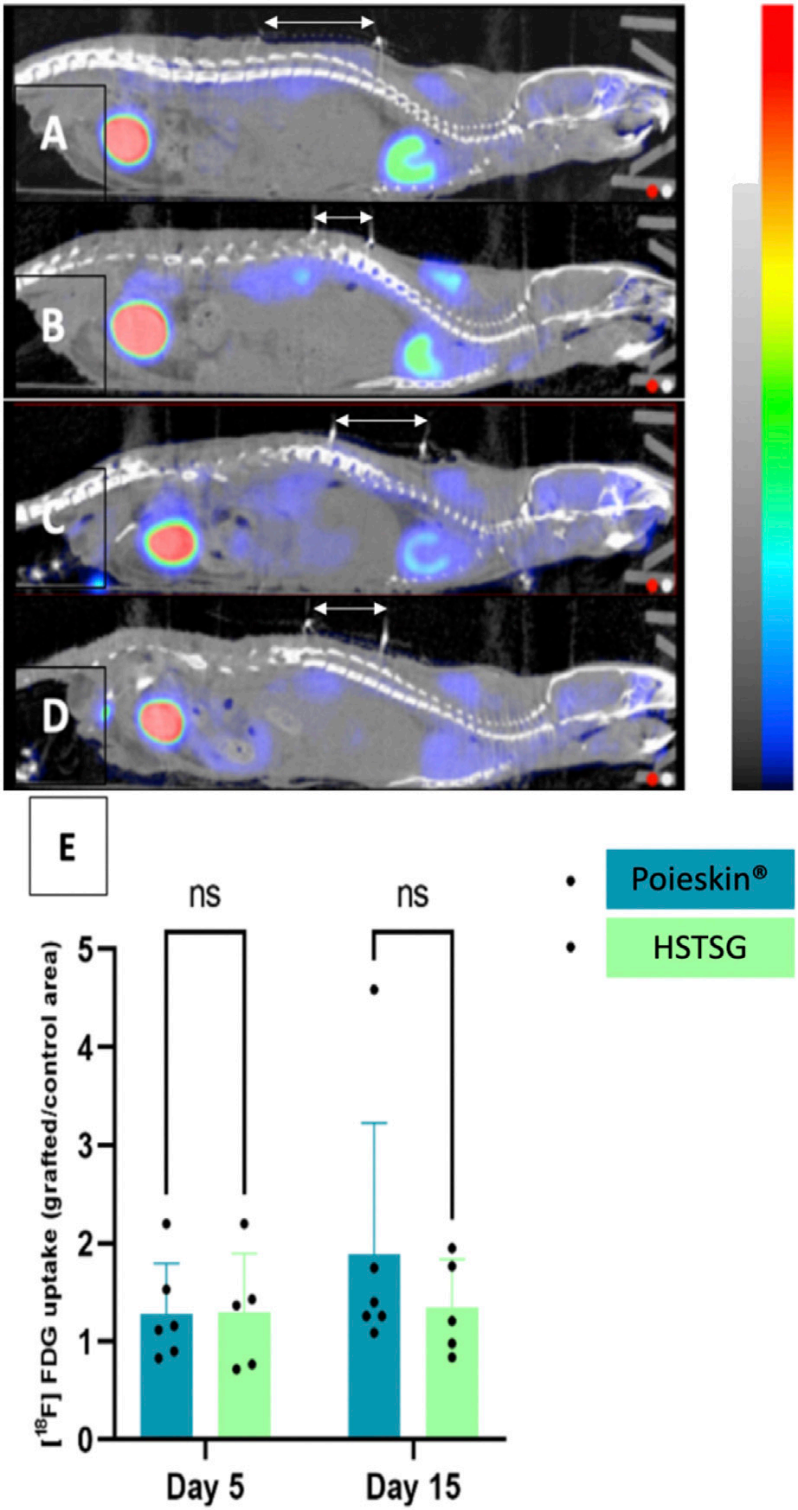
[18F]-FDG MicroPET/CT imaging (CT signal from −500 to 3,500 HU; PET signal from 0 to 8 Bq) 1 h after IV injection. Grafts are located below the white arrows ((A) Poieskin^®^ on day 5; (B) HSTSG on day 5; (C) Poieskin^®^ on day 15; (D) HSTSG on day 15; (E) metabolic activity measurements).
